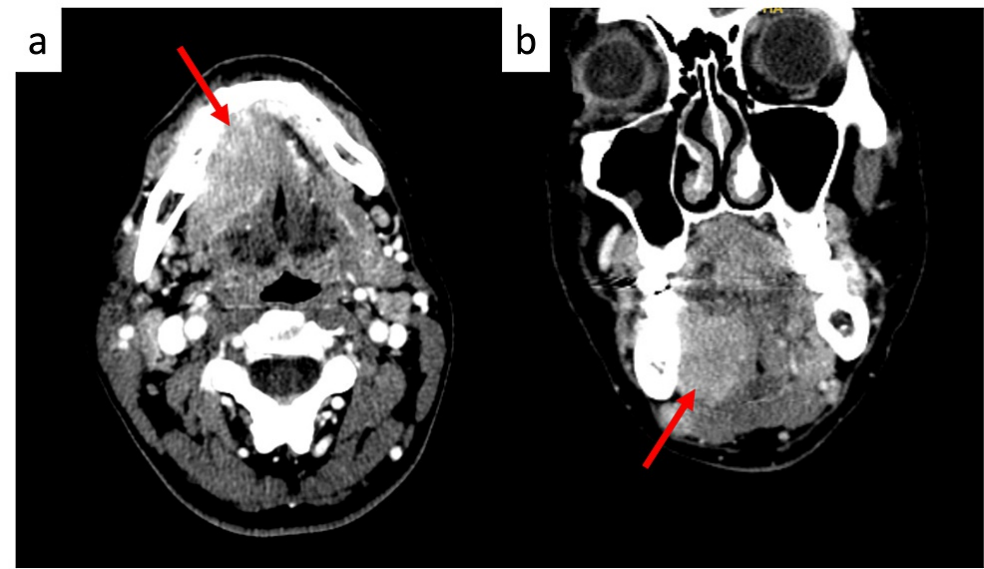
Axial (a) and coronal (b) CT head and neck images with contrast A 3.6 x 2.1 x 2.6 cm enhancing mass centered in the right aspect of the floor of the mouth (red arrows)
